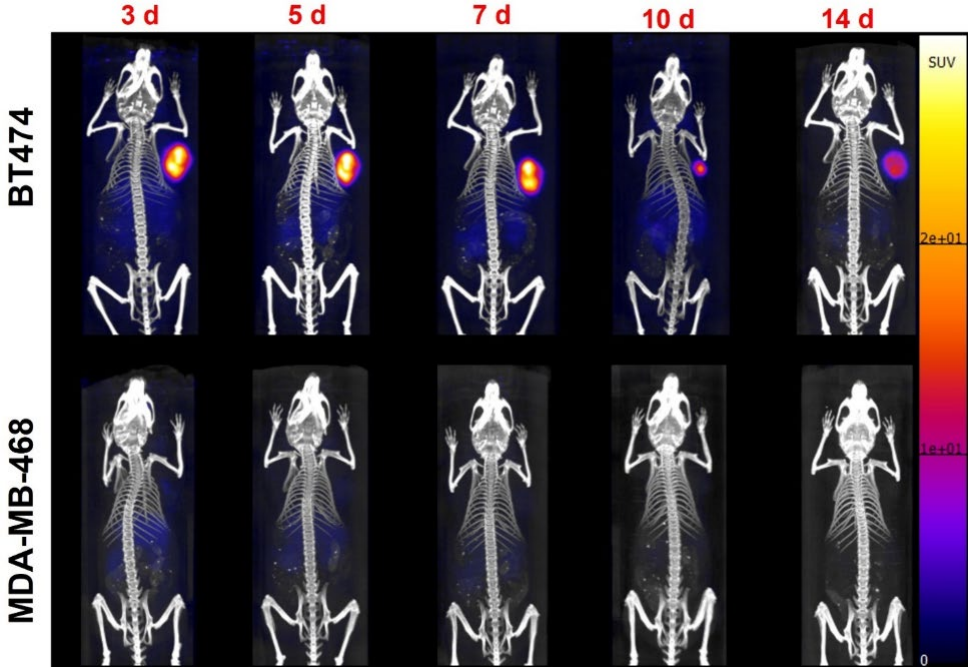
PET/CT images of [^52^Mn]Mn-Oxo-DO3A-trastuzumab in BT474 and MDA-MB-468 xenograft models showing images out to 14 d post injection.
